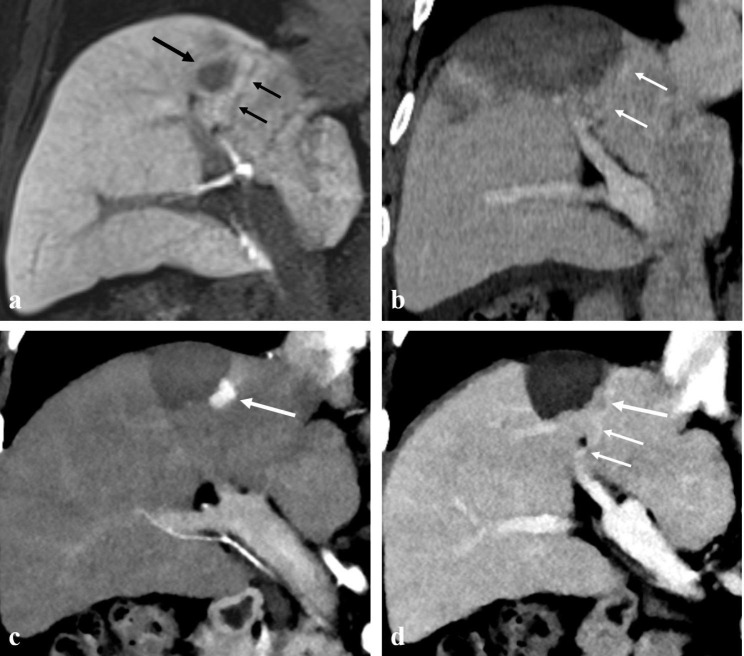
Technical failure of anatomical radiofrequency ablation in a 60-year-old man with a 2.0-cm hepatocellular carcinoma (HCC) and hepatitis B-related cirrhosis. (A) Hepatobiliary phase image of gadoxetic acid enhanced MRI shows a 2.0-cm low signal intensity HCC in segment VIII of the liver (large arrow). The 4th order portal vein branch close to the medial border of the tumor is visualized (small arrows). (B) Portal venous phase coronal reformatted image of immediate CT scan shows an insufficient medial safety margin (< 5 mm). Ablation of the 4th order portal vein branch (arrows) located near the medial border of the tumor had failed. (C) Arterial phase coronal reformatted image of 6-month follow-up CT shows a recurrent tumor with arterial enhancement at the medial aspect of the ablation defect (arrow). (D) Portal venous phase coronal reformatted image of 6-month follow-up CT shows a connection between the recurrent tumor (large arrow) and remnant 4th order portal vein branch (small arrows), which was not ablated
